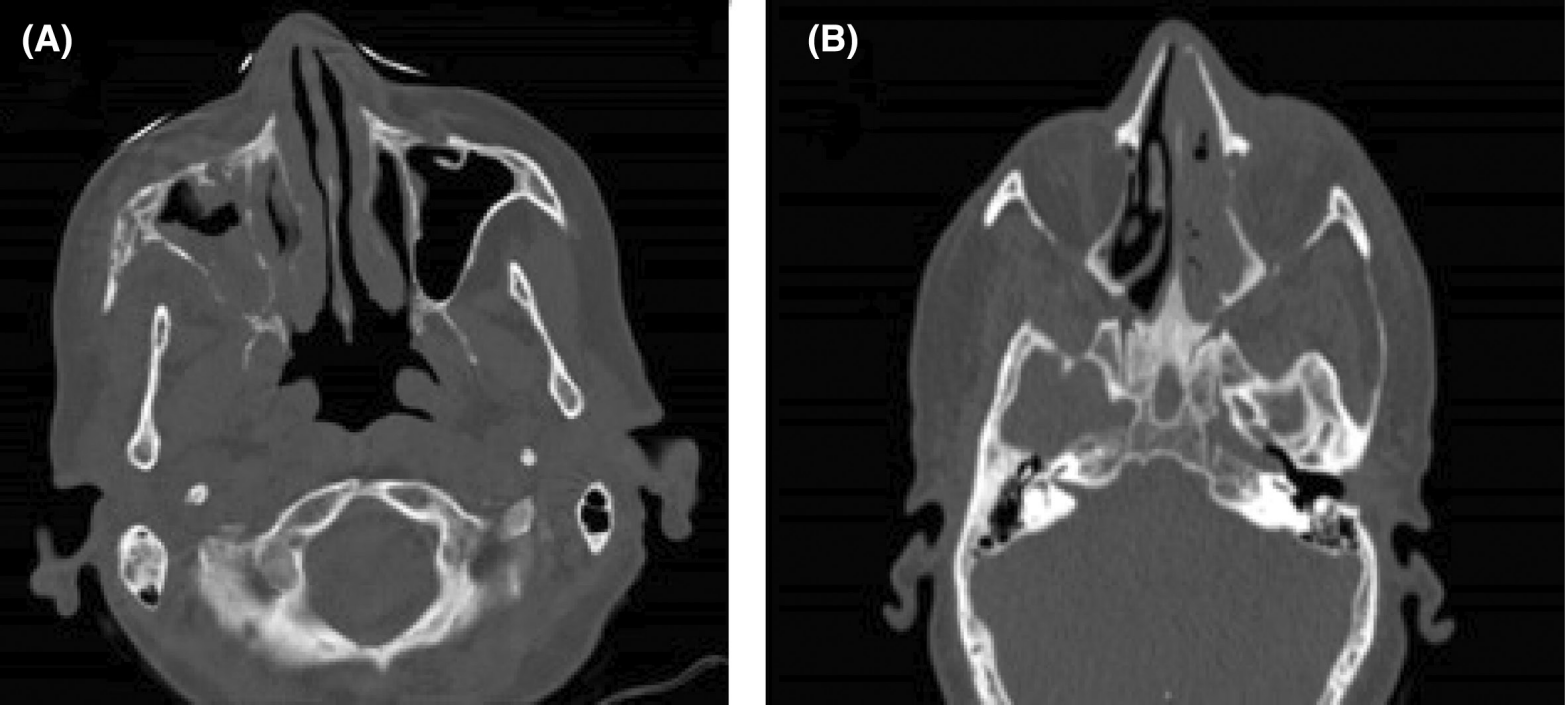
(A) Mucormycosis involvement of the right maxillary sinus and middle turbinate along with erosions of sinus walls in CT scan. (B) Opacity in left ethmoidal sinus and nasal cavity with lamina papyracea destruction and orbital involvement in CT scan.
